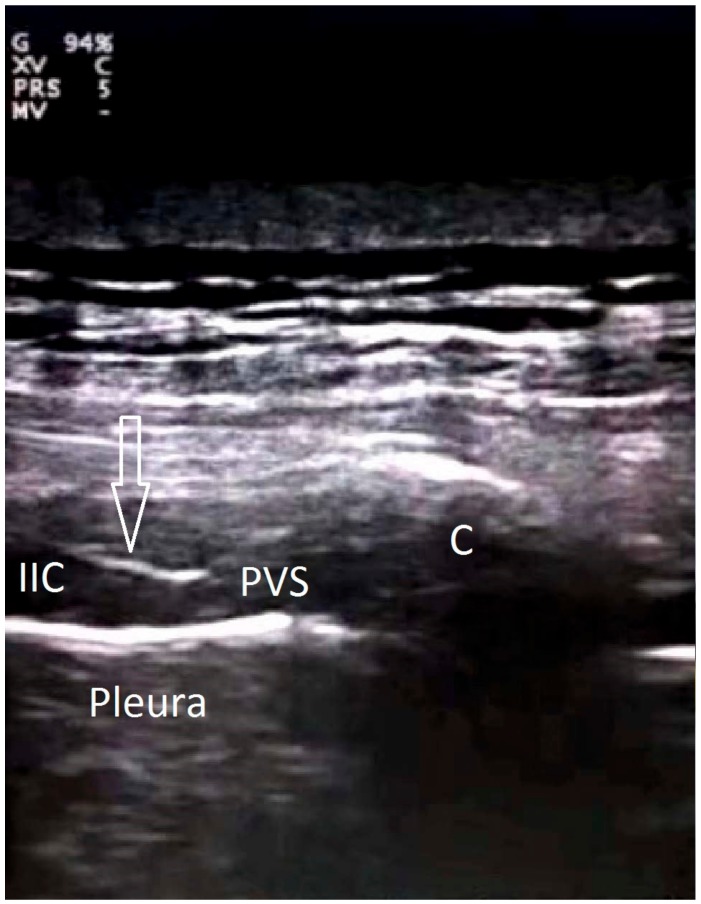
Ultrasound image showing an example injection. EIC: External intercostal muscles; IIC: internal intercostal muscles; PVS: paravertebral space; c: costa; arrows: needle.
